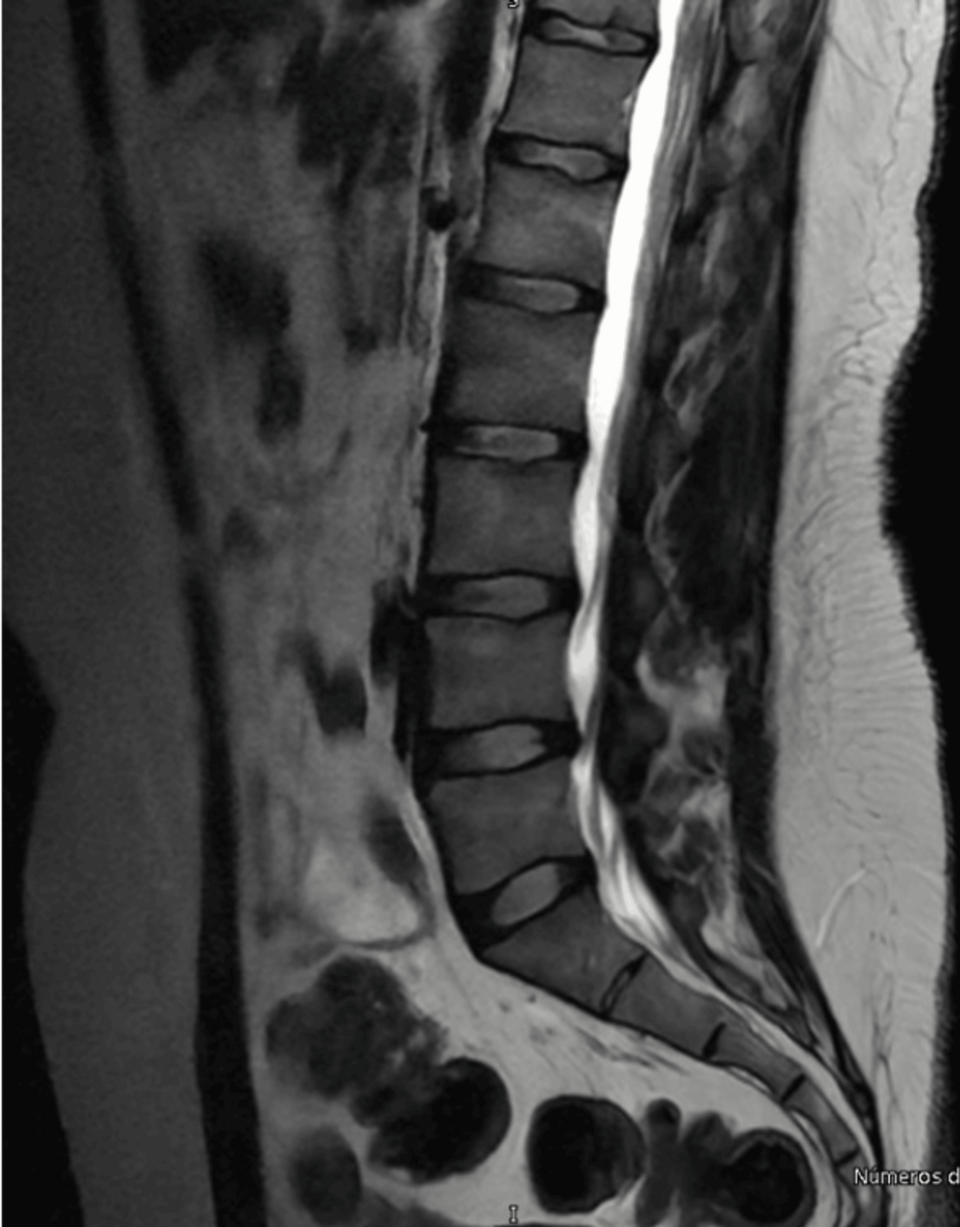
Degenerative changes noted in the lumbar spine via contrast magnetic resonance imaging (MRI) in a T2 sequence during the six-month follow-up. Sagittal section shows a L4-L5 disc protrusion (arrows). Adjacent intervertebral discs are observed without evidence of dehydration or significant degenerative changes. In the lower region, fat infiltration of paraspinal muscles is observed.
